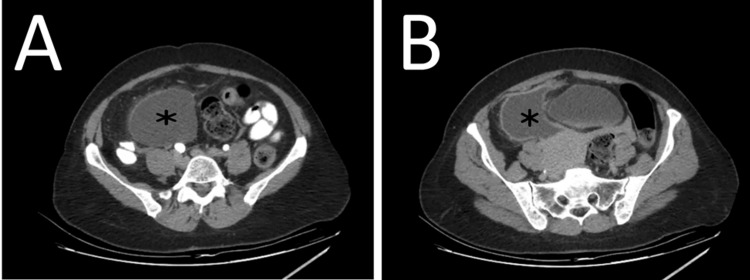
(A,B): A contrast-enhanced computed tomography (CECT) scan showing a well-defined 10-cm right infraumbilical hypodense mass showing a cystic density with peripheral enhancement (asterisks).
